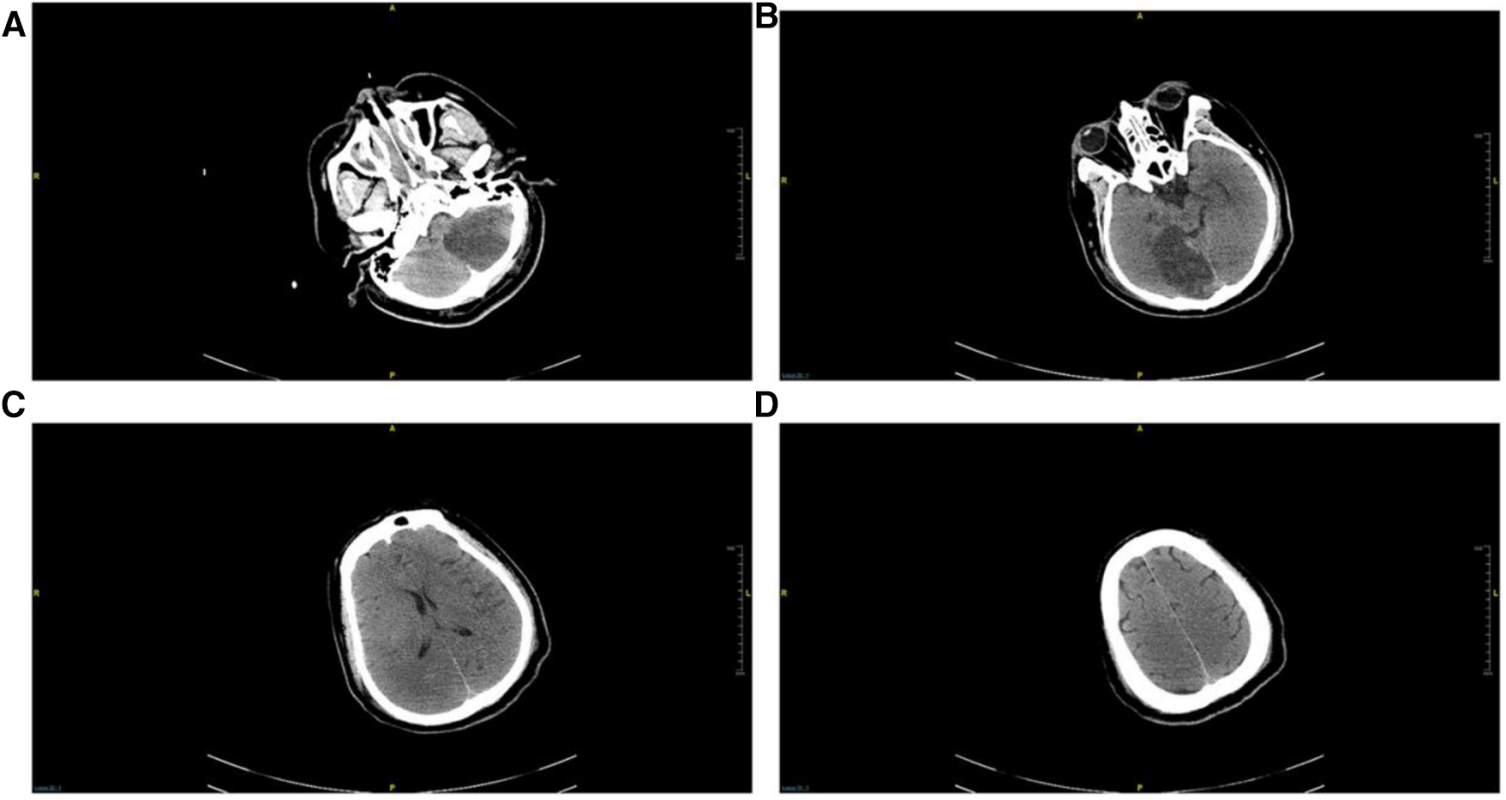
Repeated head CT on the 4th day after admission. (A) Horizontal cross-section of the petrous part of the temporal bone, (B) horizontal cross-section of the pons, (C) coronal radiata section, (D) superior cross-section of the frontal lobe. Ischemic lesions were identified in the left cerebellum and right occipital lobe, while no discernible lesions were detected in the remaining regions.
